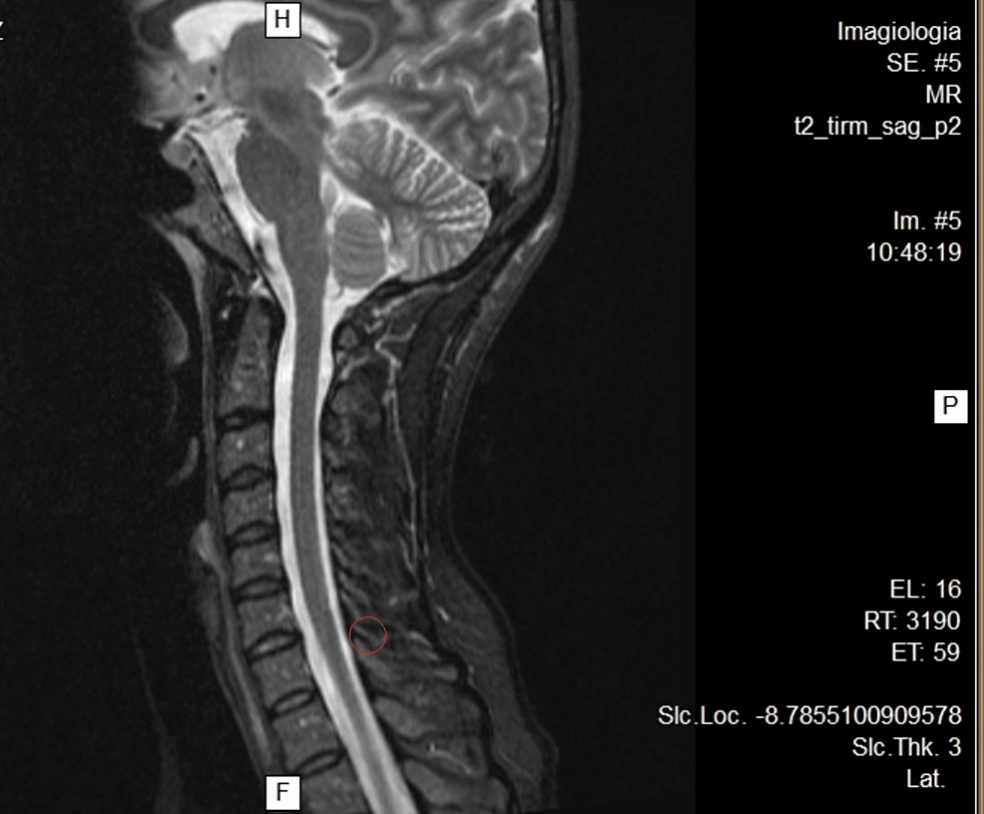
Cervical MRI. Incipient foraminal asymmetry in C4-C5
